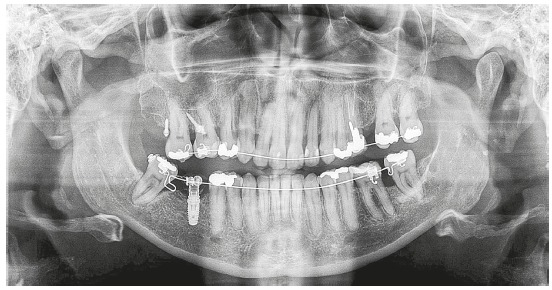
Panoramic radiograph after placement of miniscrews for intrusion of tooth 16 and endosseous implant for uprighting of tooth 48.
